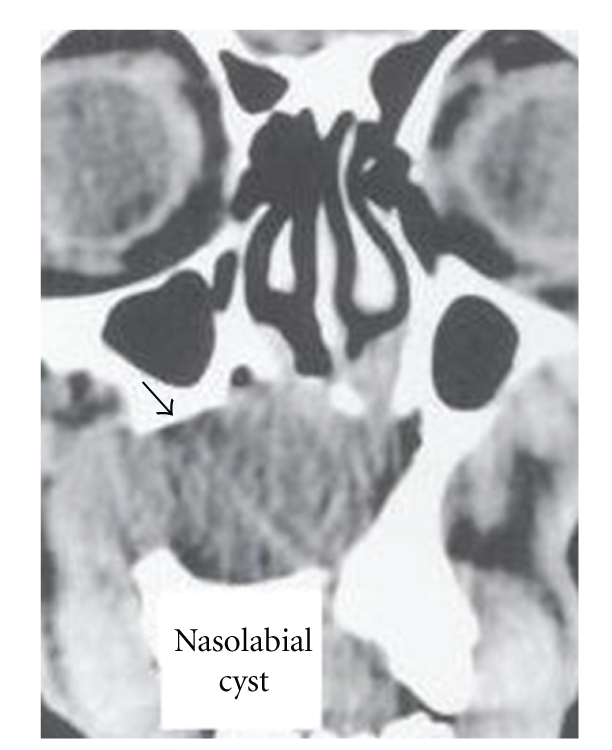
CT scan of the lesion (dark arrow indicates the lesion).
